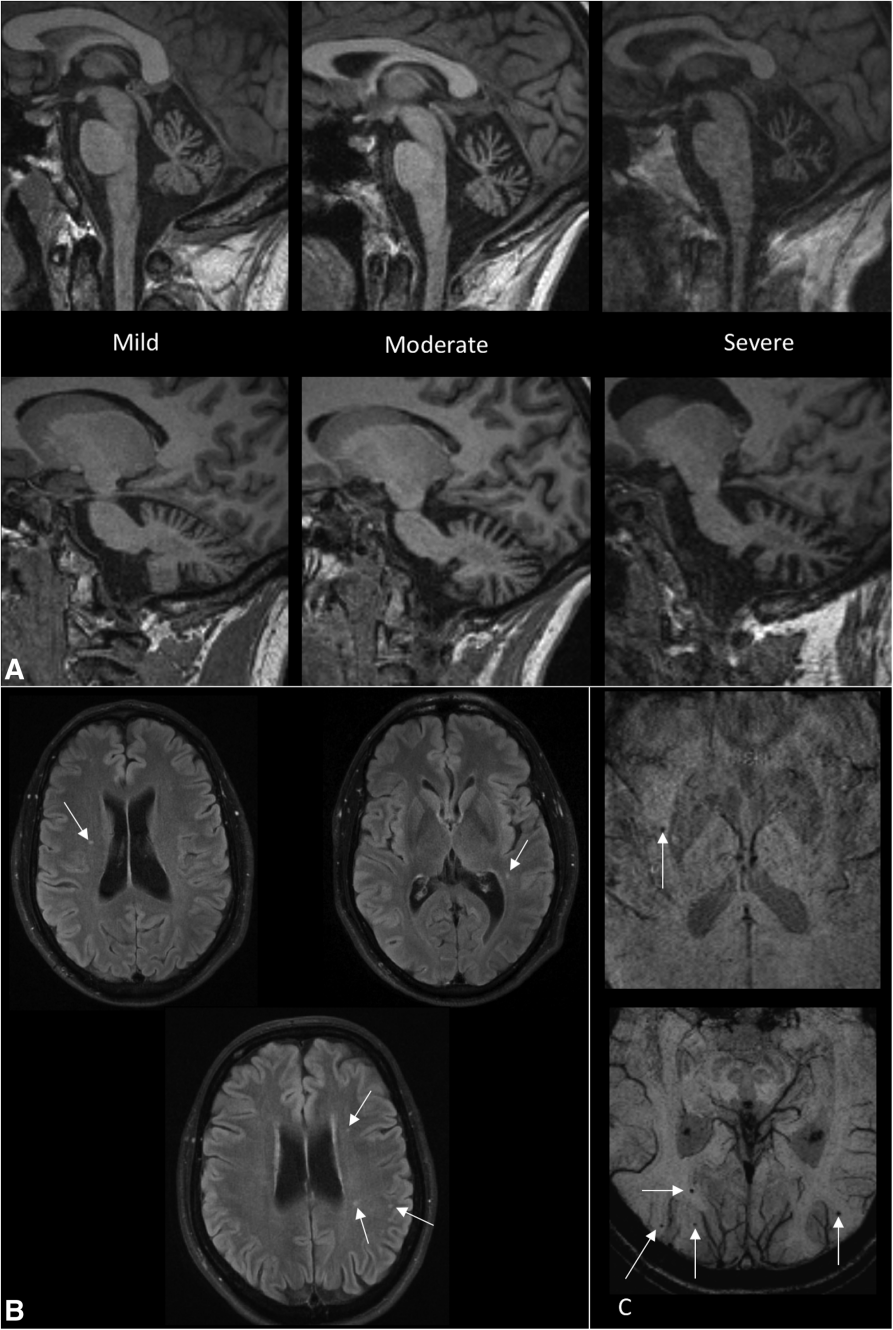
(A) MRI scans showing examples of mild, moderate and severe cerebellar atrophy. The top row shows midline images to assess vermian atrophy and the bottom row shows parasagittal images for assessing hemispheric atrophy. (B) MRI scans showing examples of white matter lesions. (C) MRI scans showing microbleeds. MRI = magnetic resonance imaging.
